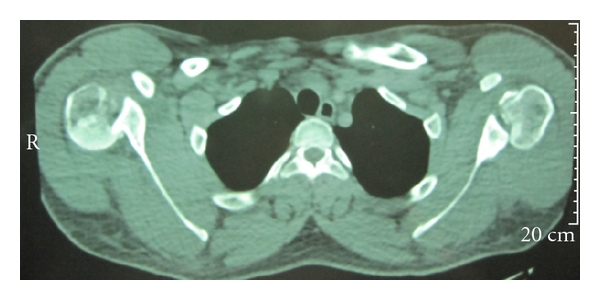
Axial CT scan view reveals bilateral shoulder posterior dislocation with medium defect of articular surfaces (50% in the right, 30% in the left).
